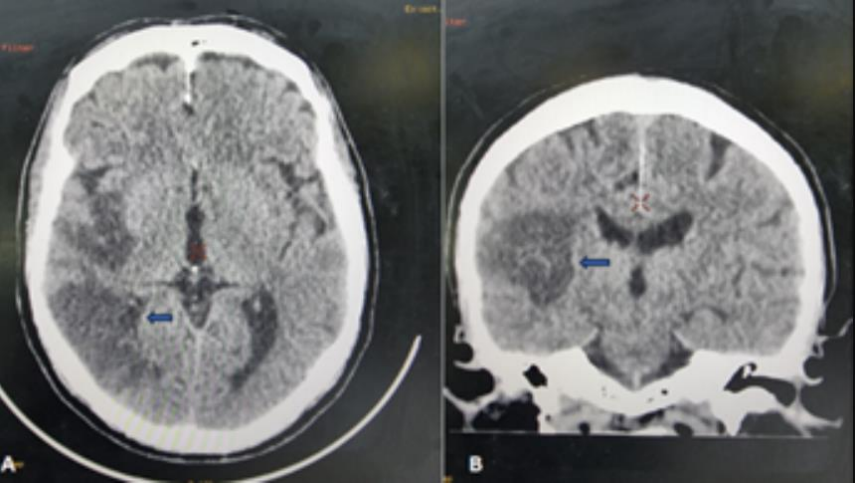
computed tomography scan in axial (A) and coronal (B) sections revealing a right temporo-parietal hypodensity, consistent with ischemic stroke in the Sylvian territory; arrows point to the area of ischemia
